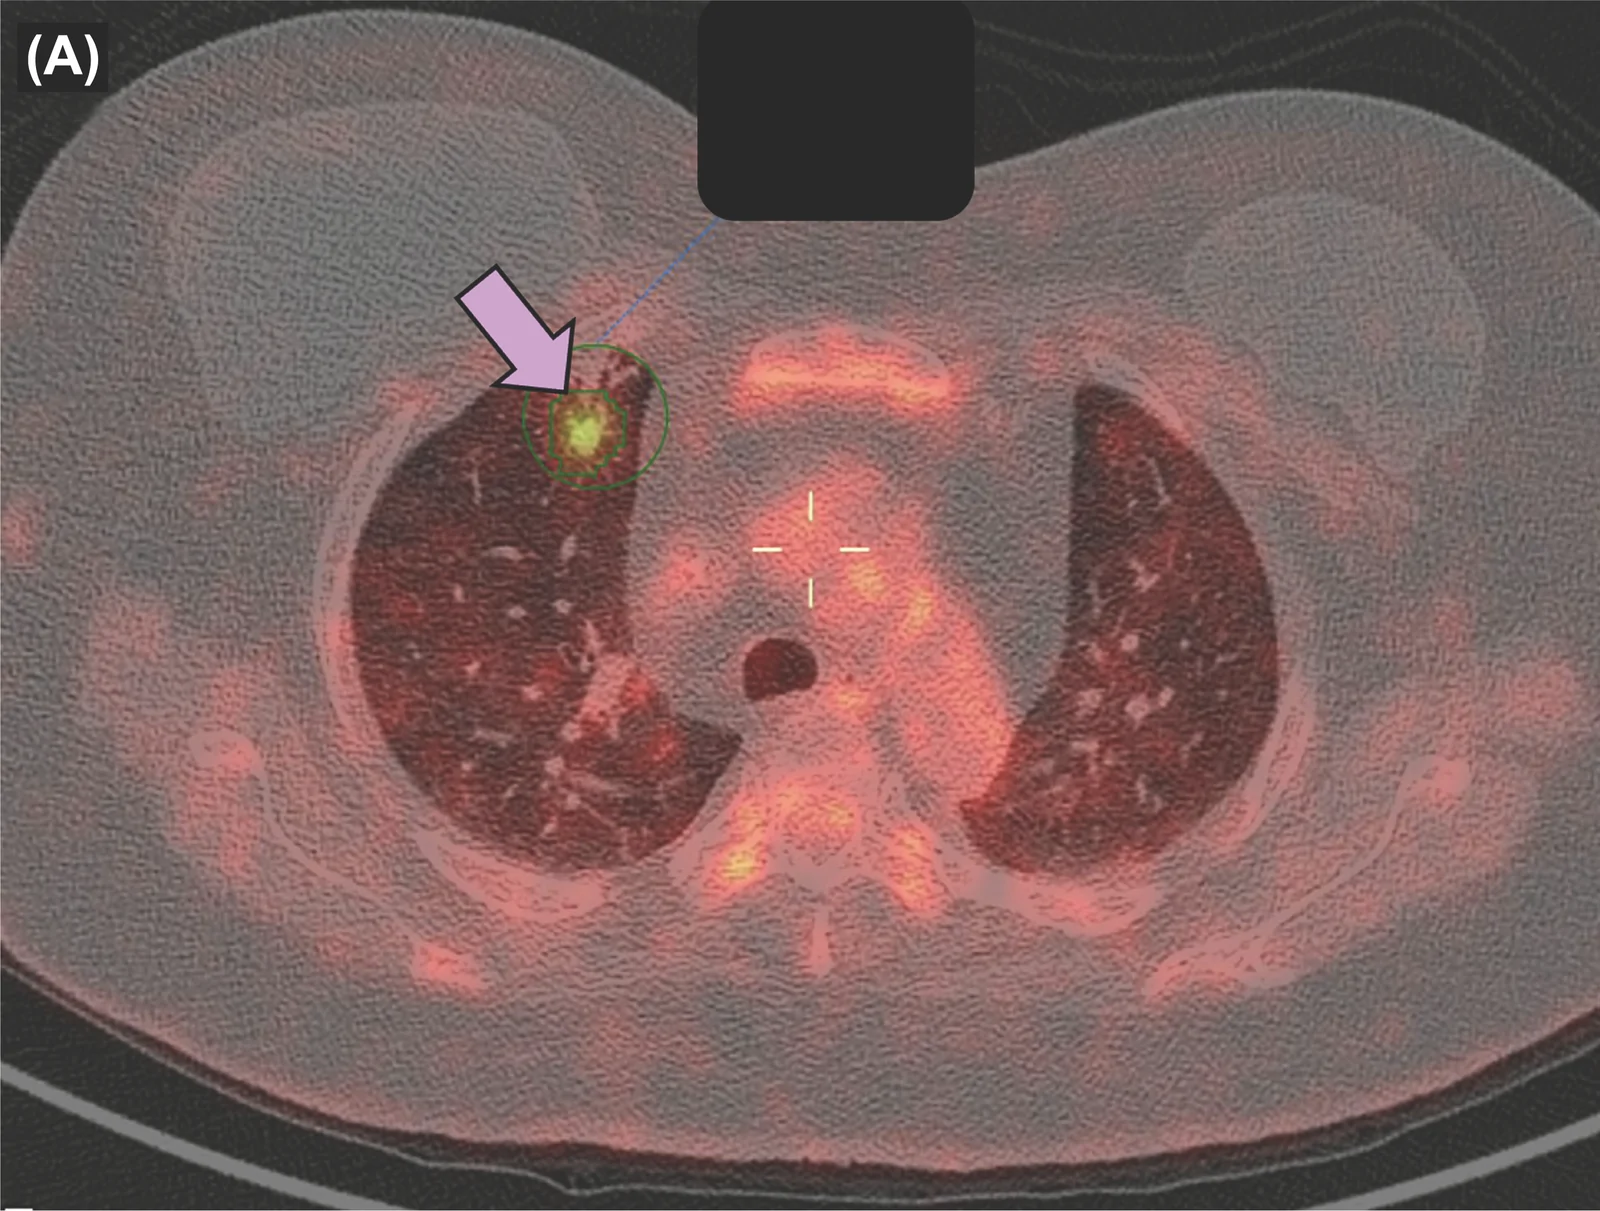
18F-FDG PET/CT. Hypermetabolic spiculated pulmonary nodule in the right upper lobe with SUVmax 4.0 (purple arrow) and local architectural distortion. Abbreviations: FDG, fluorodeoxyglucose; PET/CT, positron emission tomography/computed tomography; SUVmax, maximum standardized uptake value.
